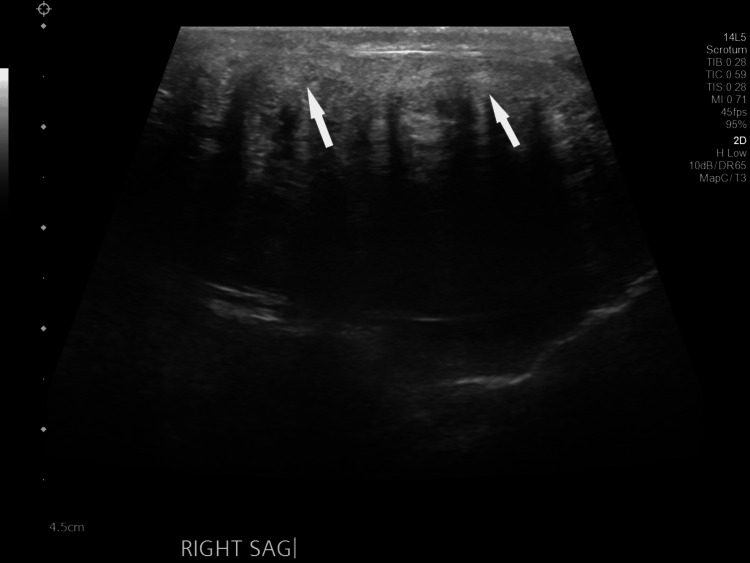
Grey-scale sagittal ultrasound image of the right testicle demonstrating shadowing masses consistent with testicular adrenal rest tumors in this patient with a history of congenital adrenal hyperplasia (white arrows).
